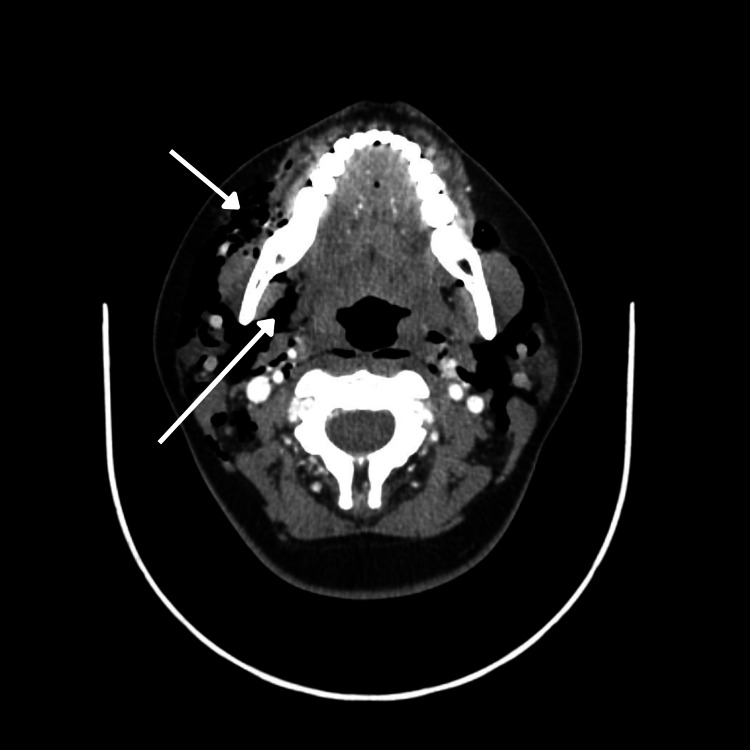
Axial contrast-enhanced CT at the level of the mandible and oropharynx Gas (arrows) dissects the bilateral masticator, buccal, parapharyngeal, and submandibular spaces. CT: computed tomography
